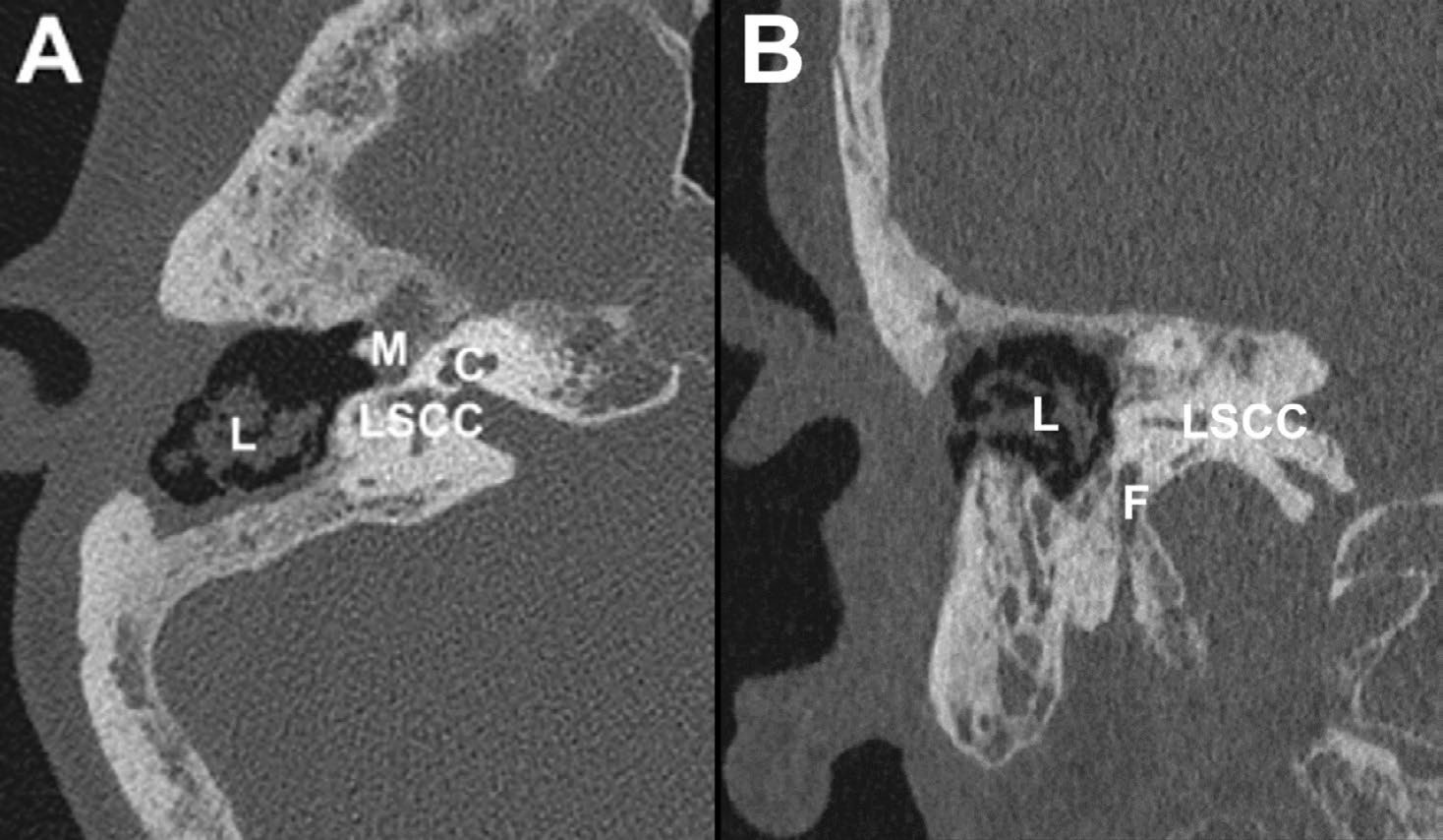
Computed tomography of the temporal bone in axial (A) and coronal (B) planes showing multiple soft‐tissue densities compatible with larvae occupying the radical mastoid cavity. Normal anatomical landmarks are indicated: C, cochlea; F, facial nerve; L, larvae; LSCC, lateral semicircular canal; M, malleus.
